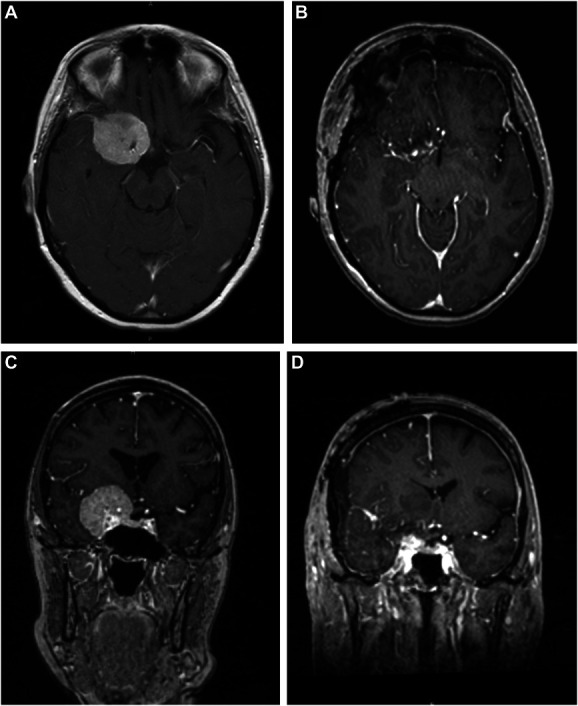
Axial view of T1-weighted MRI with contrast demonstrating a right sphenoid wing meningioma A, before and B, after resection. Coronal view of T1-weighted MRI C, before and D, after resection demonstrating a near-total resection, with small residual tumor encasing the right supraclinoid internal carotid artery at its intracranial origin.
